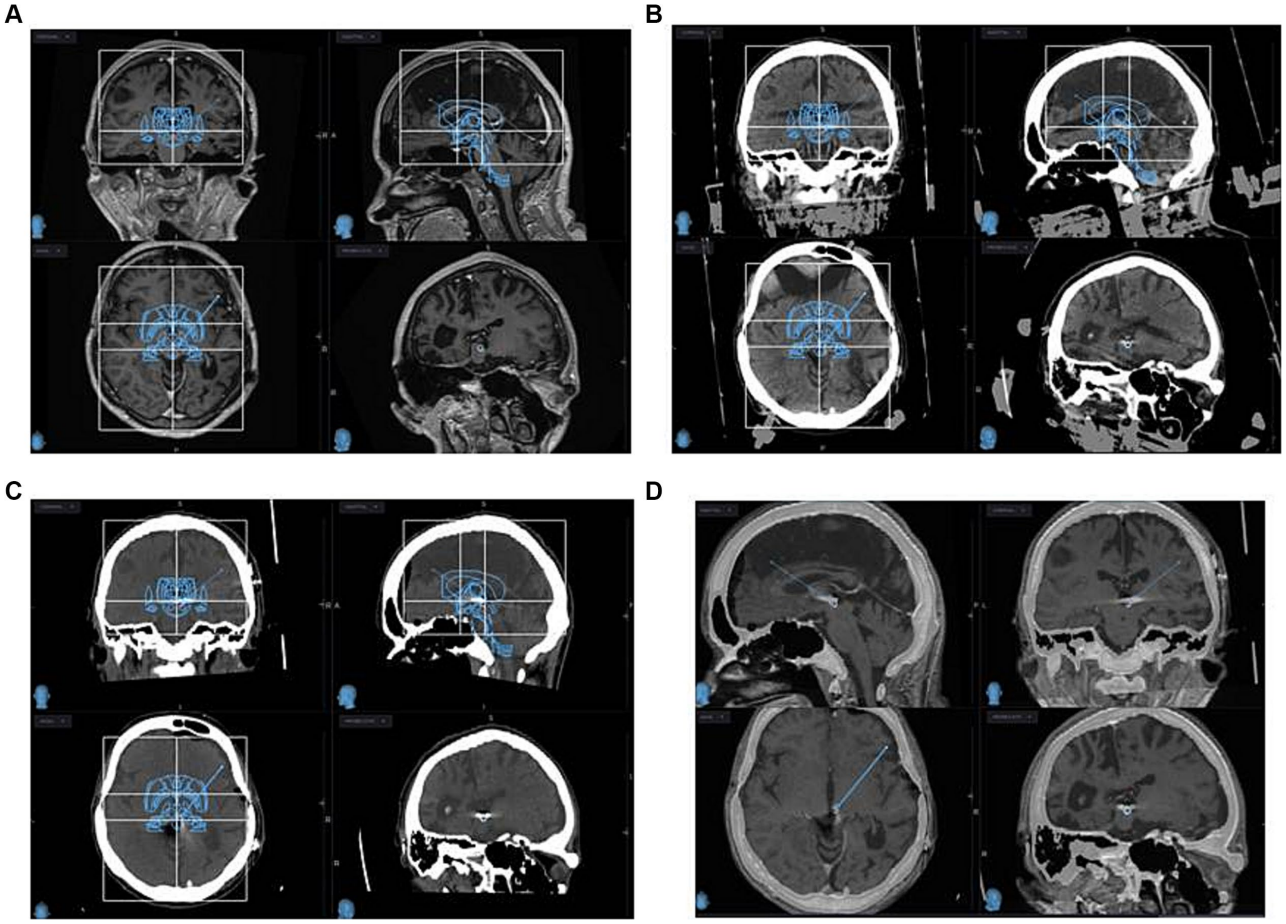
MRI (A) and CT with stereotactic frame (B) planning images of PAG/PVG DBS implantation are depicted. Post-implantation CT (C) and MRI (D) images are depicted. Infused Schaltenbrand Wahren atlas and Talairach grids are visualized for indirect identification of PAG-PVG (A–C) at coronal, sagittal, and axial projections.
